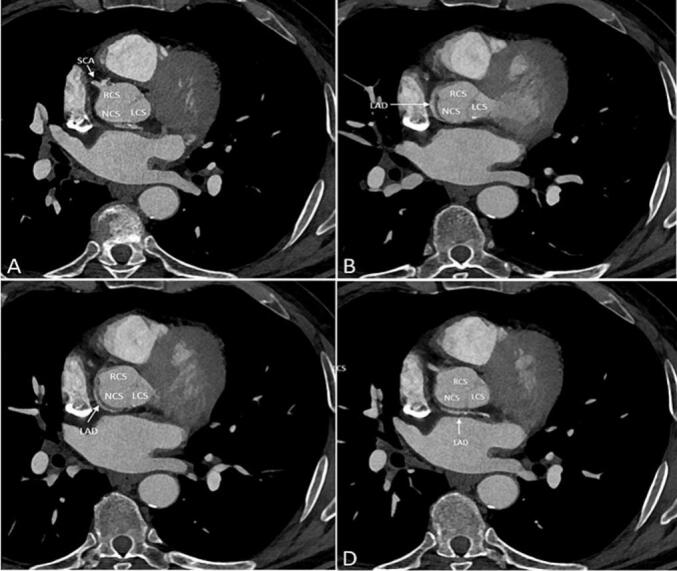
Coronary CT Angiography showing single coronary artery (SCA) (A) and a posterior course around the aorta of left anterior descending artery (LAD) (B-C).
